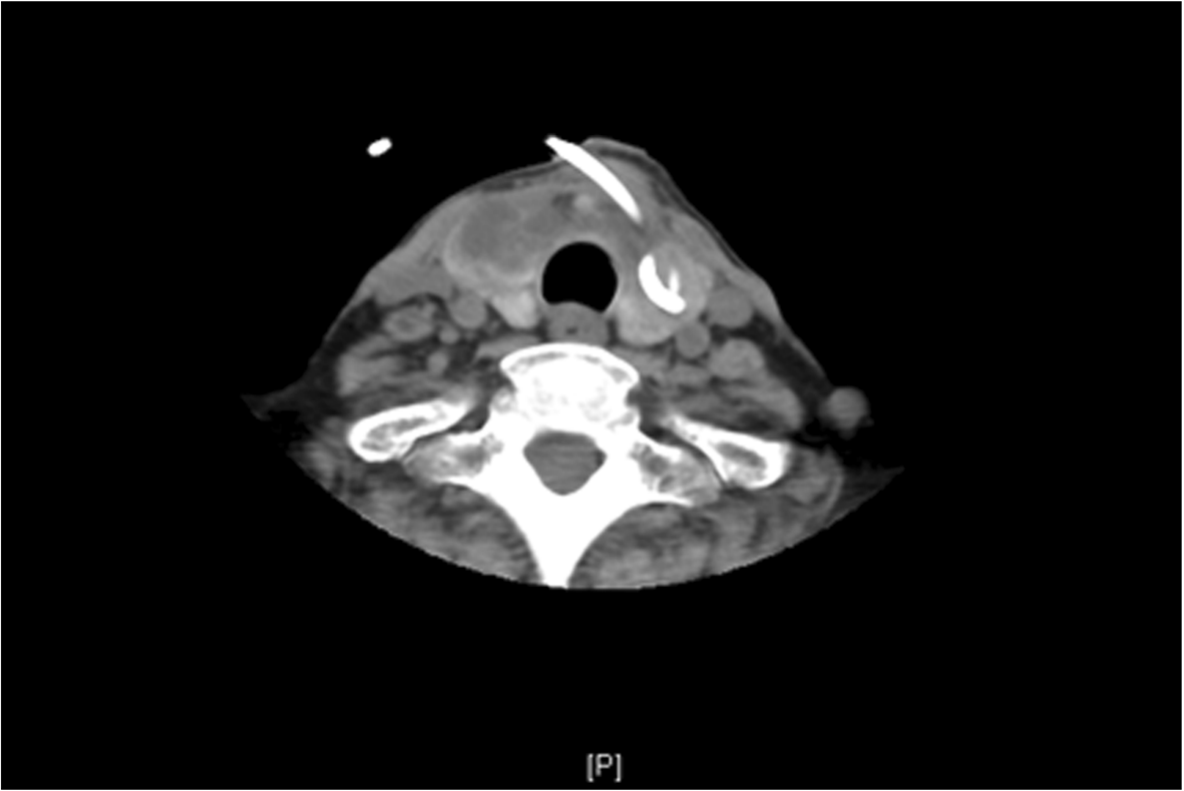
Neck computed tomography revealed effective drainage of the left lobe of the thyroid gland while abscesses remained in the right lobe
